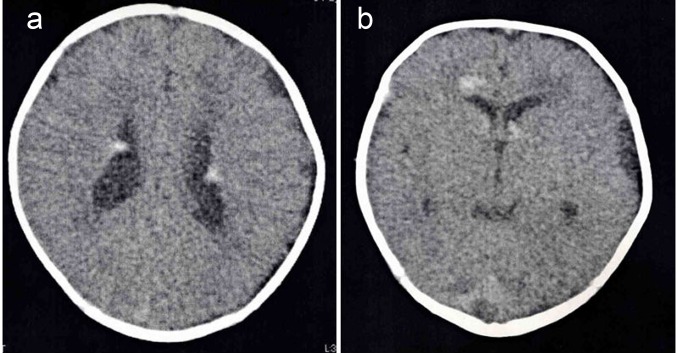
CT of the head. There were multiple sclerosed regions at the wall of lateral ventricle (a) and foramen of Monro (b).
